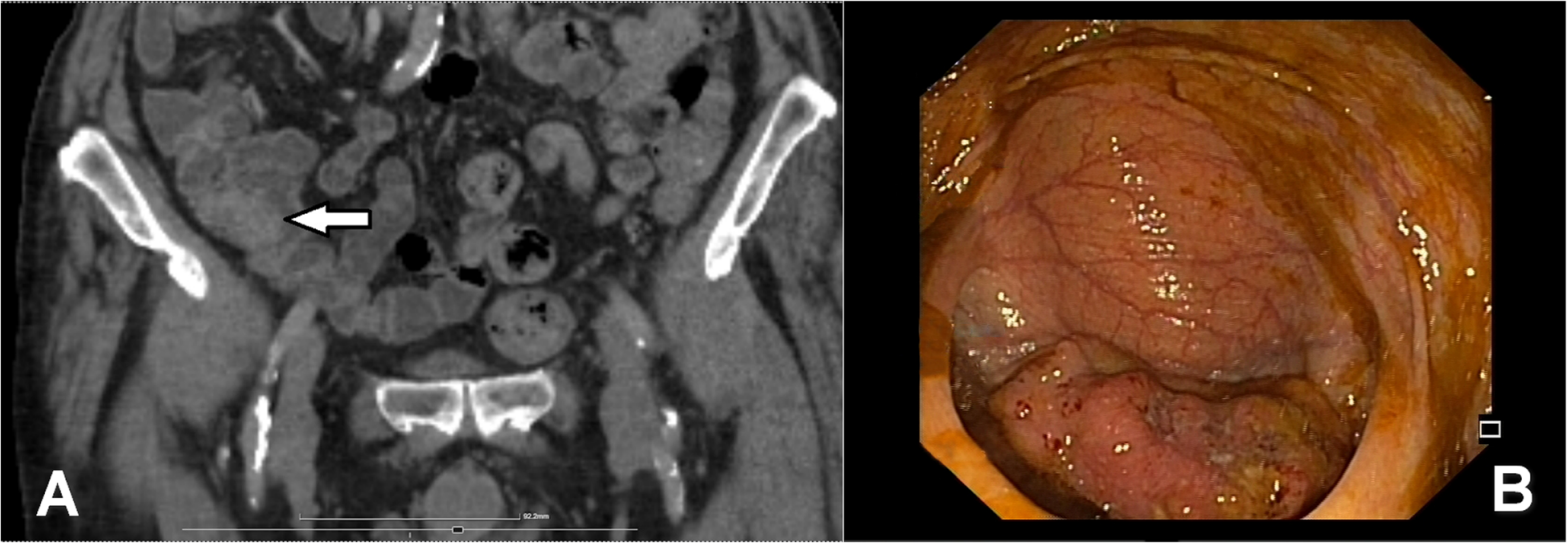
a, b Abdominal computer tomography (CT) and colonoscopic image of cecal cancer
